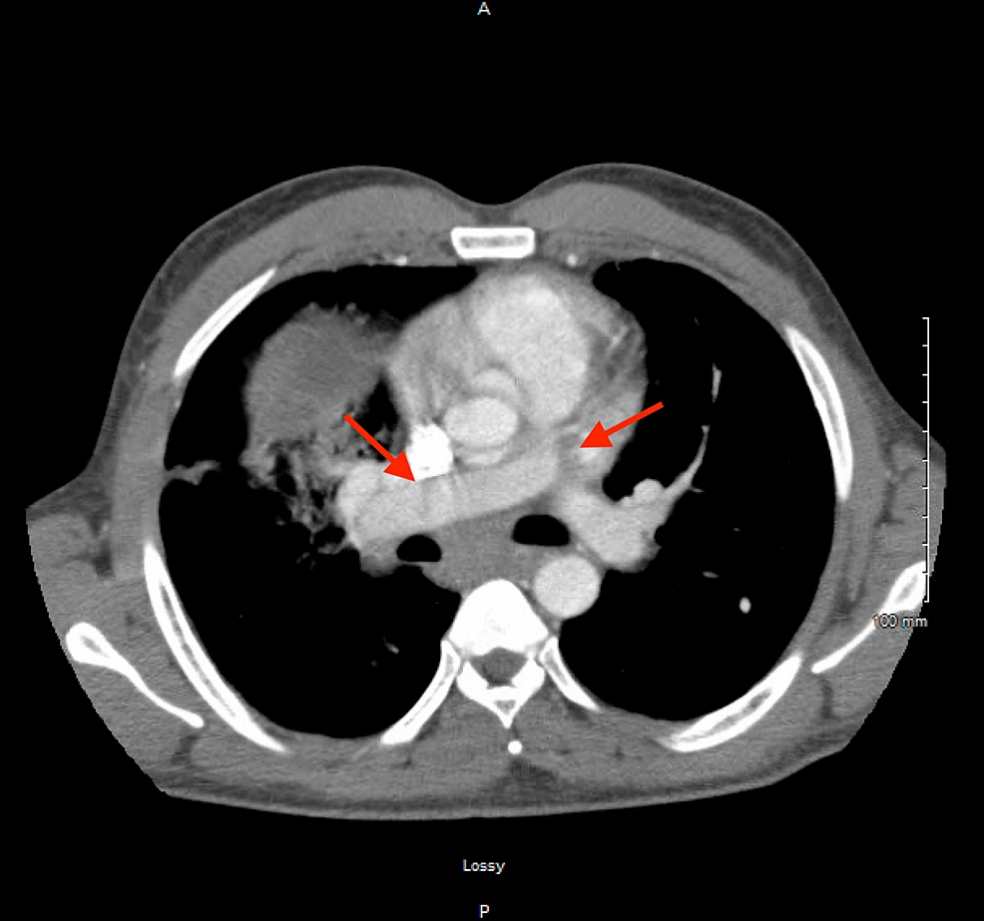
CTPA showing bilateral pulmonary emboli (red arrows). CTPA, computed tomography pulmonary angiography
